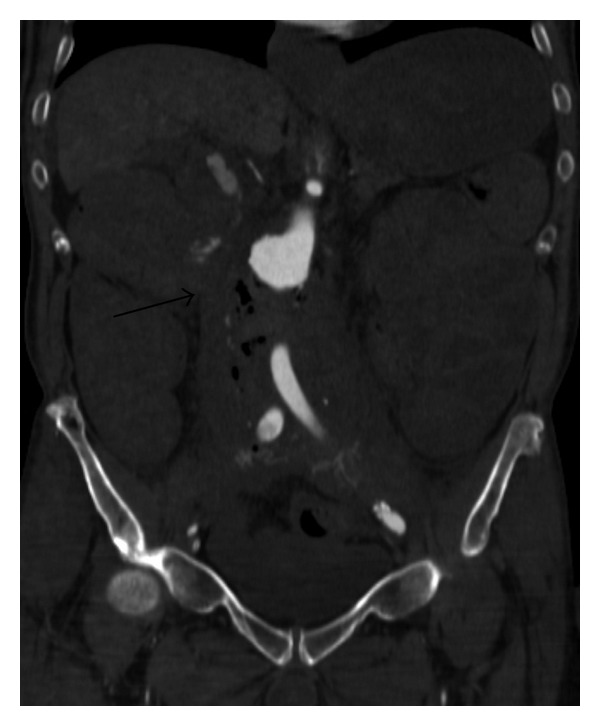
Coronal contrast-enhanced CT scan of AEF.
